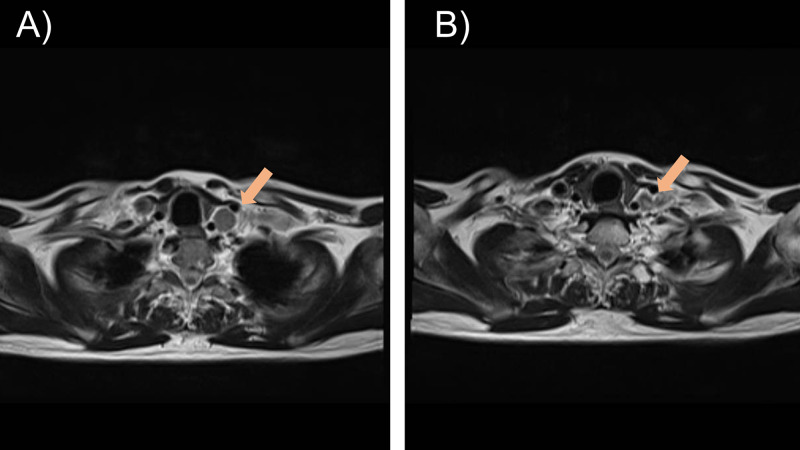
Magnetic resonance imaging Magnetic resonance imaging showing a significant reduction in the size of the sub/supra-clavicular lymph nodes A) before radiotherapy and B) after radiotherapy
